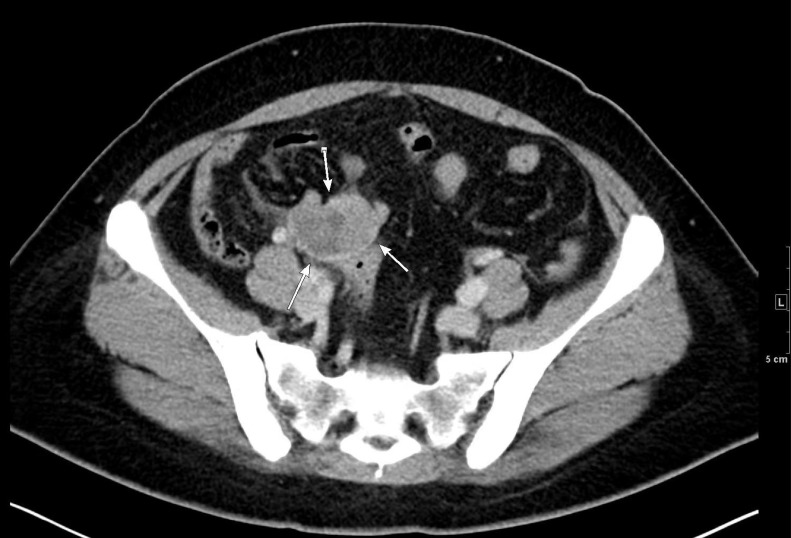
The pelvic CT revealed a 5 cm multi-locular solid-cystic mass in the pelvic cavity (the white arrows indicated the border of mass).
